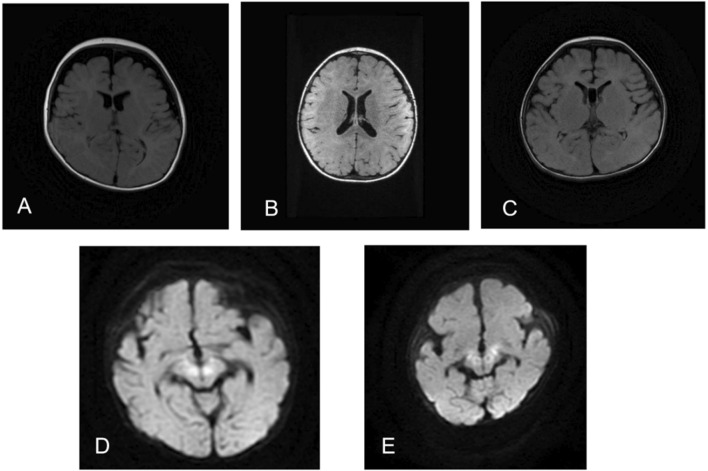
Magnetic resonance imaging (MRI) details of patients: (A) patient #1 at 8 months of age with widening of the extracerebral space (FLAIR); (B) patient #2 at 1 year of age with normal MRI (FLAIR); (C) patient #3 at 1 year of age with widening of the hyaline septum (FLAIR); (D) patient #4 at 2 months of age with high diffusion-weighted imaging (DWI) signals in the brainstem and basal ganglia; (E) patient #5 at 1 month of age with high DWI signals in the brainstem and basal ganglia.
